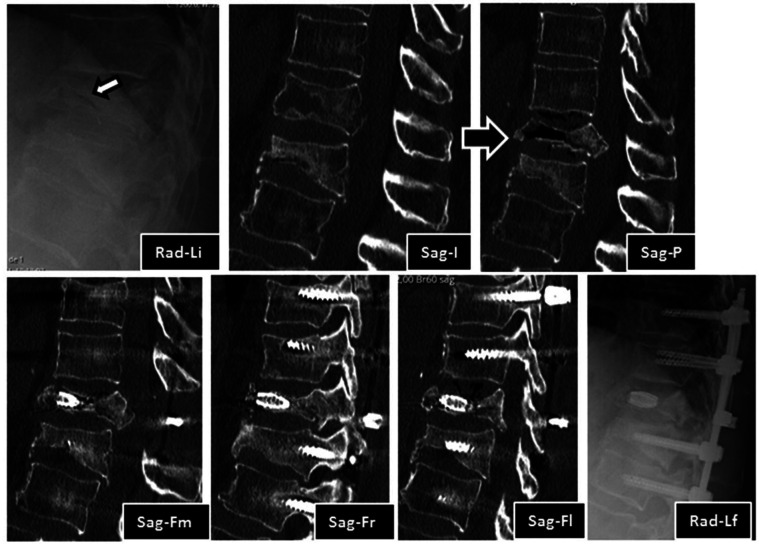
Imaging evolution of the clinical case in lateral view radiography images and sagittal CT sections: Rad-Li—initial radiograph in lateral view, showing T12 and L1 vertebral body flatenning and extensive bone destruction at T12 (arrow); Sag-I—initial median sagittal view of computed tomography, showing acute T12 fracture and old L1 fracture. Note the extensive bone trabeculae destruction at anterior half of the vertebral body; Sag-*P*—Median sagittal view of tomography, showing T12 vertebral body pseudarthrosis with a large anterior intravertebral cleft and marked posterior wall retropulsion; Sag-Fm—Median sagittal view of CT at 1 year after surgery, showing T12 vertebral body pseudarthrosis filled with stent and signs of T12 laminectomy; Sag-Fr—Right parasagittal view of CT at 1 year after surgery, showing the right stent filled with bone graft and the right pedicular screws, note the pseudarthrosis healing; Sag-Fl—Left parasagittal view of CT at 1 year after surgery, showing the left stent filled with bone graft and the right pedicular screws. Note the pseudarthrosis healing; Rad-Lf—Final radiograph in lateral view at 2 years after surgery, showing T12 stents, adjacent pedicle screws, rods and crosslink.
